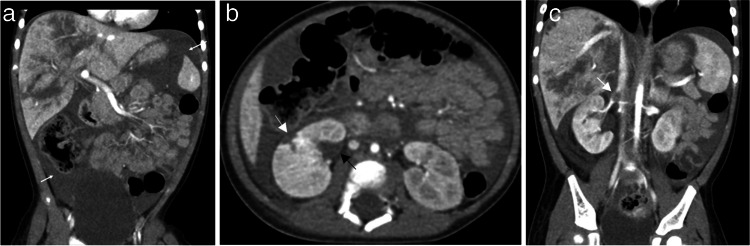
An 18-month-old male presented with lethargy and markedly elevated liver enzymes. Caregiver confessed to multiple blows to the epigastrium. a Coronal image of a post-contrast CT abdomen and pelvis in portal venous phase demonstrates extensive liver lacerations and a large volume complex peritoneal fluid (*white arrows*) with a higher density than the bladder. b Axial CT image from the same study shows two separate renal lacerations in the upper pole of the right kidney (*white and black arrows*). c Coronal image from the same study shows a post-traumatic right renal vein thrombus (*white arrow*)
